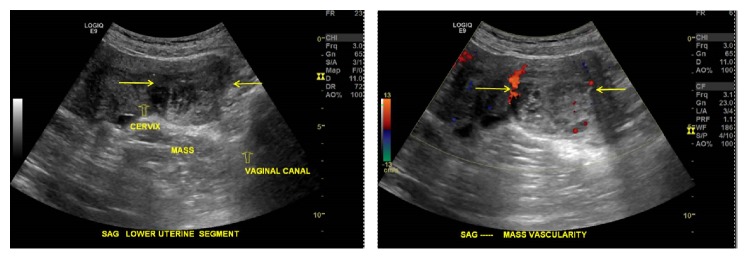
Transvaginal ultrasound. (a) Gray scale image shows a mixed echogenicity mass (arrows) with small hypoechoic cystic areas (3.0 MHz) 10 cm. (b) Color Doppler image shows minimal vascularity. Magnitude: 3 MHz.
